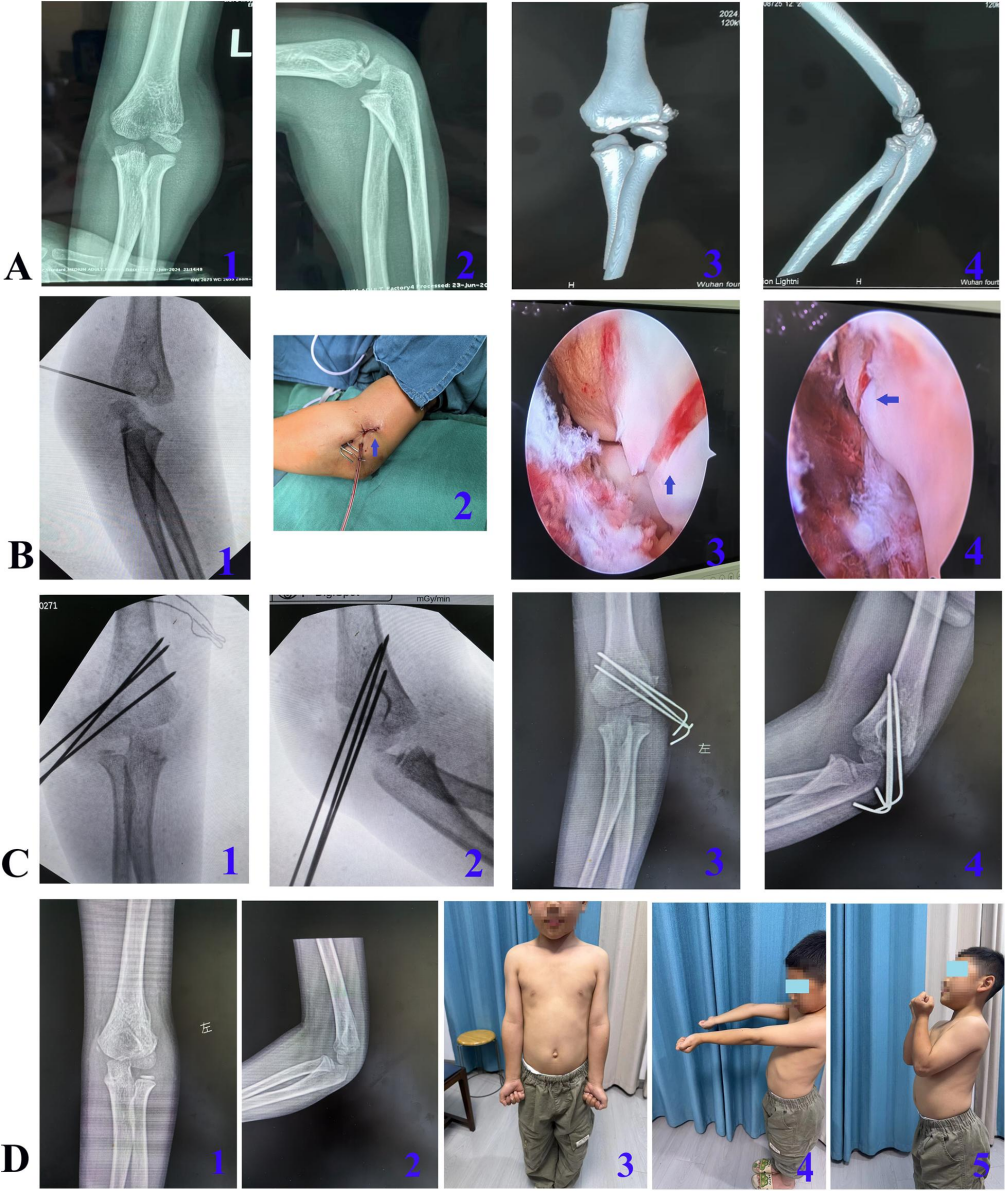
Representative case 2. A 7-year-and-6-month-old boy with a left lateral humeral condyle fracture caused by a fall. A: Preoperative imaging, including x-rays (1, 2) and CT scans (3, 4), showing a Jakob type II fracture. B: 1, Fluoroscopic localization of the fracture Line due to elbow swelling; 2, postoperative appearance of the local incision; 3 and 4, arthroscopic views before and after reduction, showing restoration of an even articular surface and a fracture gap of <2 mm. C: 1 and 2, intraoperative fluoroscopy images; 3 and 4, x-ray images at 1 month postoperatively. D: 1 and 2, x-ray images at 3 months postoperatively, showing fracture union; 3, 4, and 5, functional photographs at 6 months postoperatively, demonstrating no significant difference in the appearance of the bilateral elbow joints, with a 7° loss of extension in the affected elbow compared to the healthy side.
